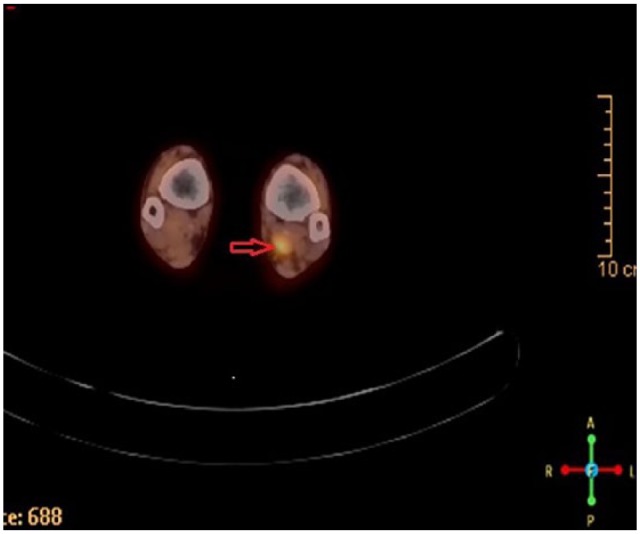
PET/CT shows hypermetabolic focus in the soft tissues of the posterior left medial ankle (arrow).
